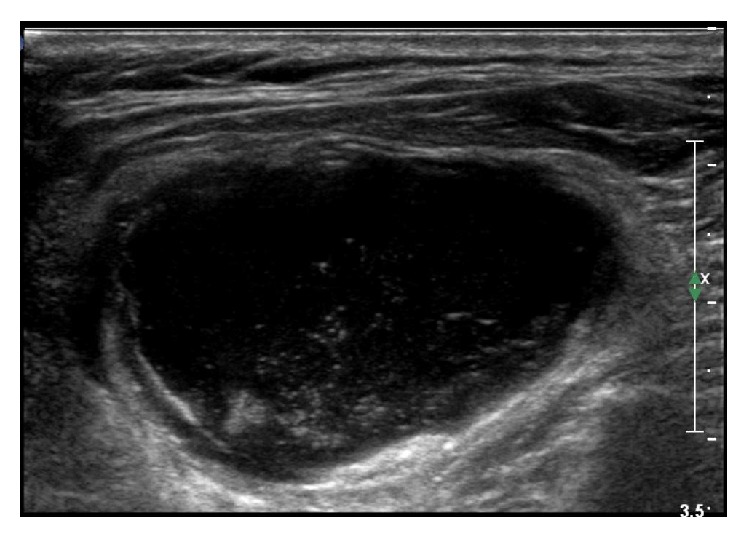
Ultrasonography showing a large heterogeneously echoic mass.
